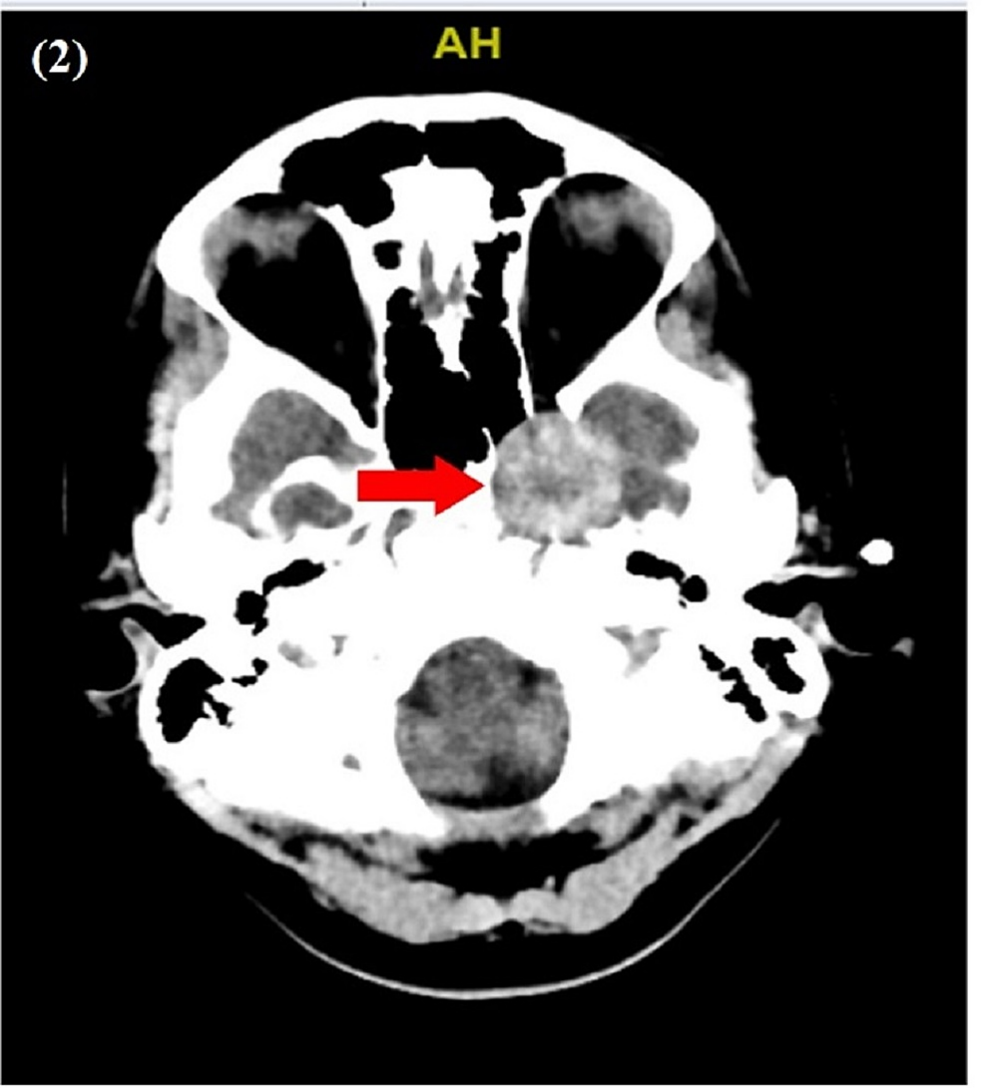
CT brain without contrast: arrow showing a mixed-density mass measuring 2.7 x 2.6 cm, which is seen in the medial aspect of the left middle cranial fossa adjacent to the left temporal lobe suggesting an intracranial neoplasm
